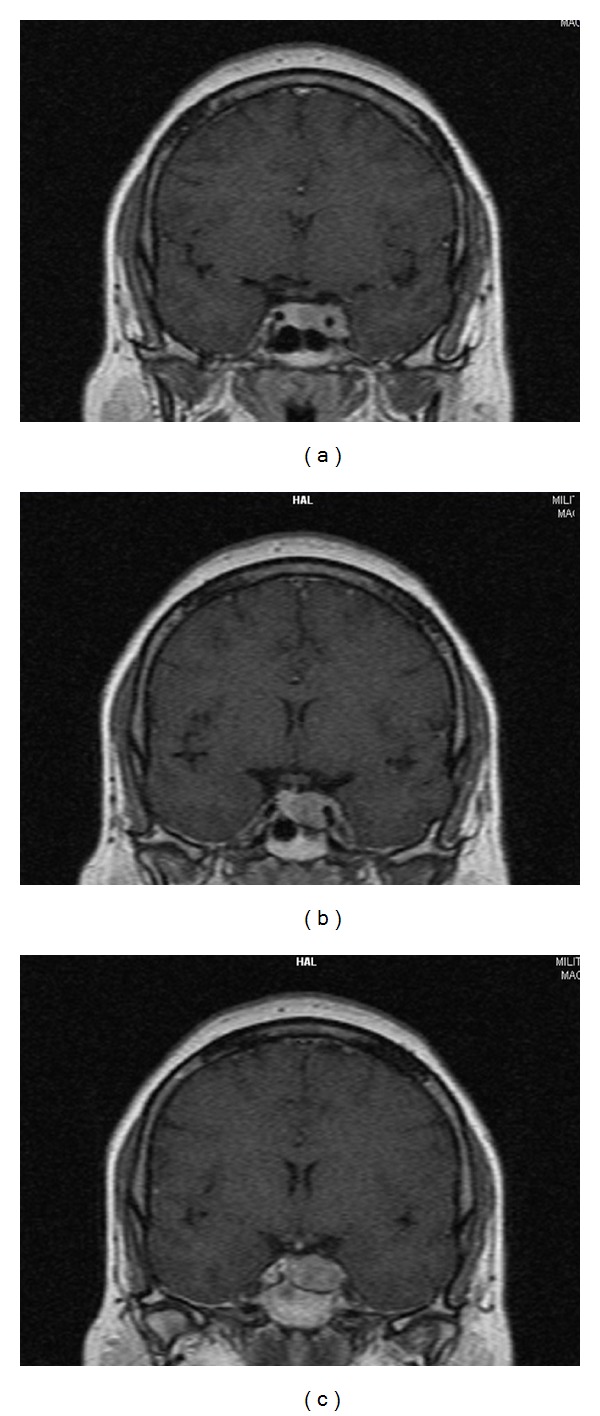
Postcontrast T1 weighted MRI at the time of diagnosis: coronal sections visualizing pituitary macroadenoma (21 × 13 mm) with the left cavernous sinus invasion.
